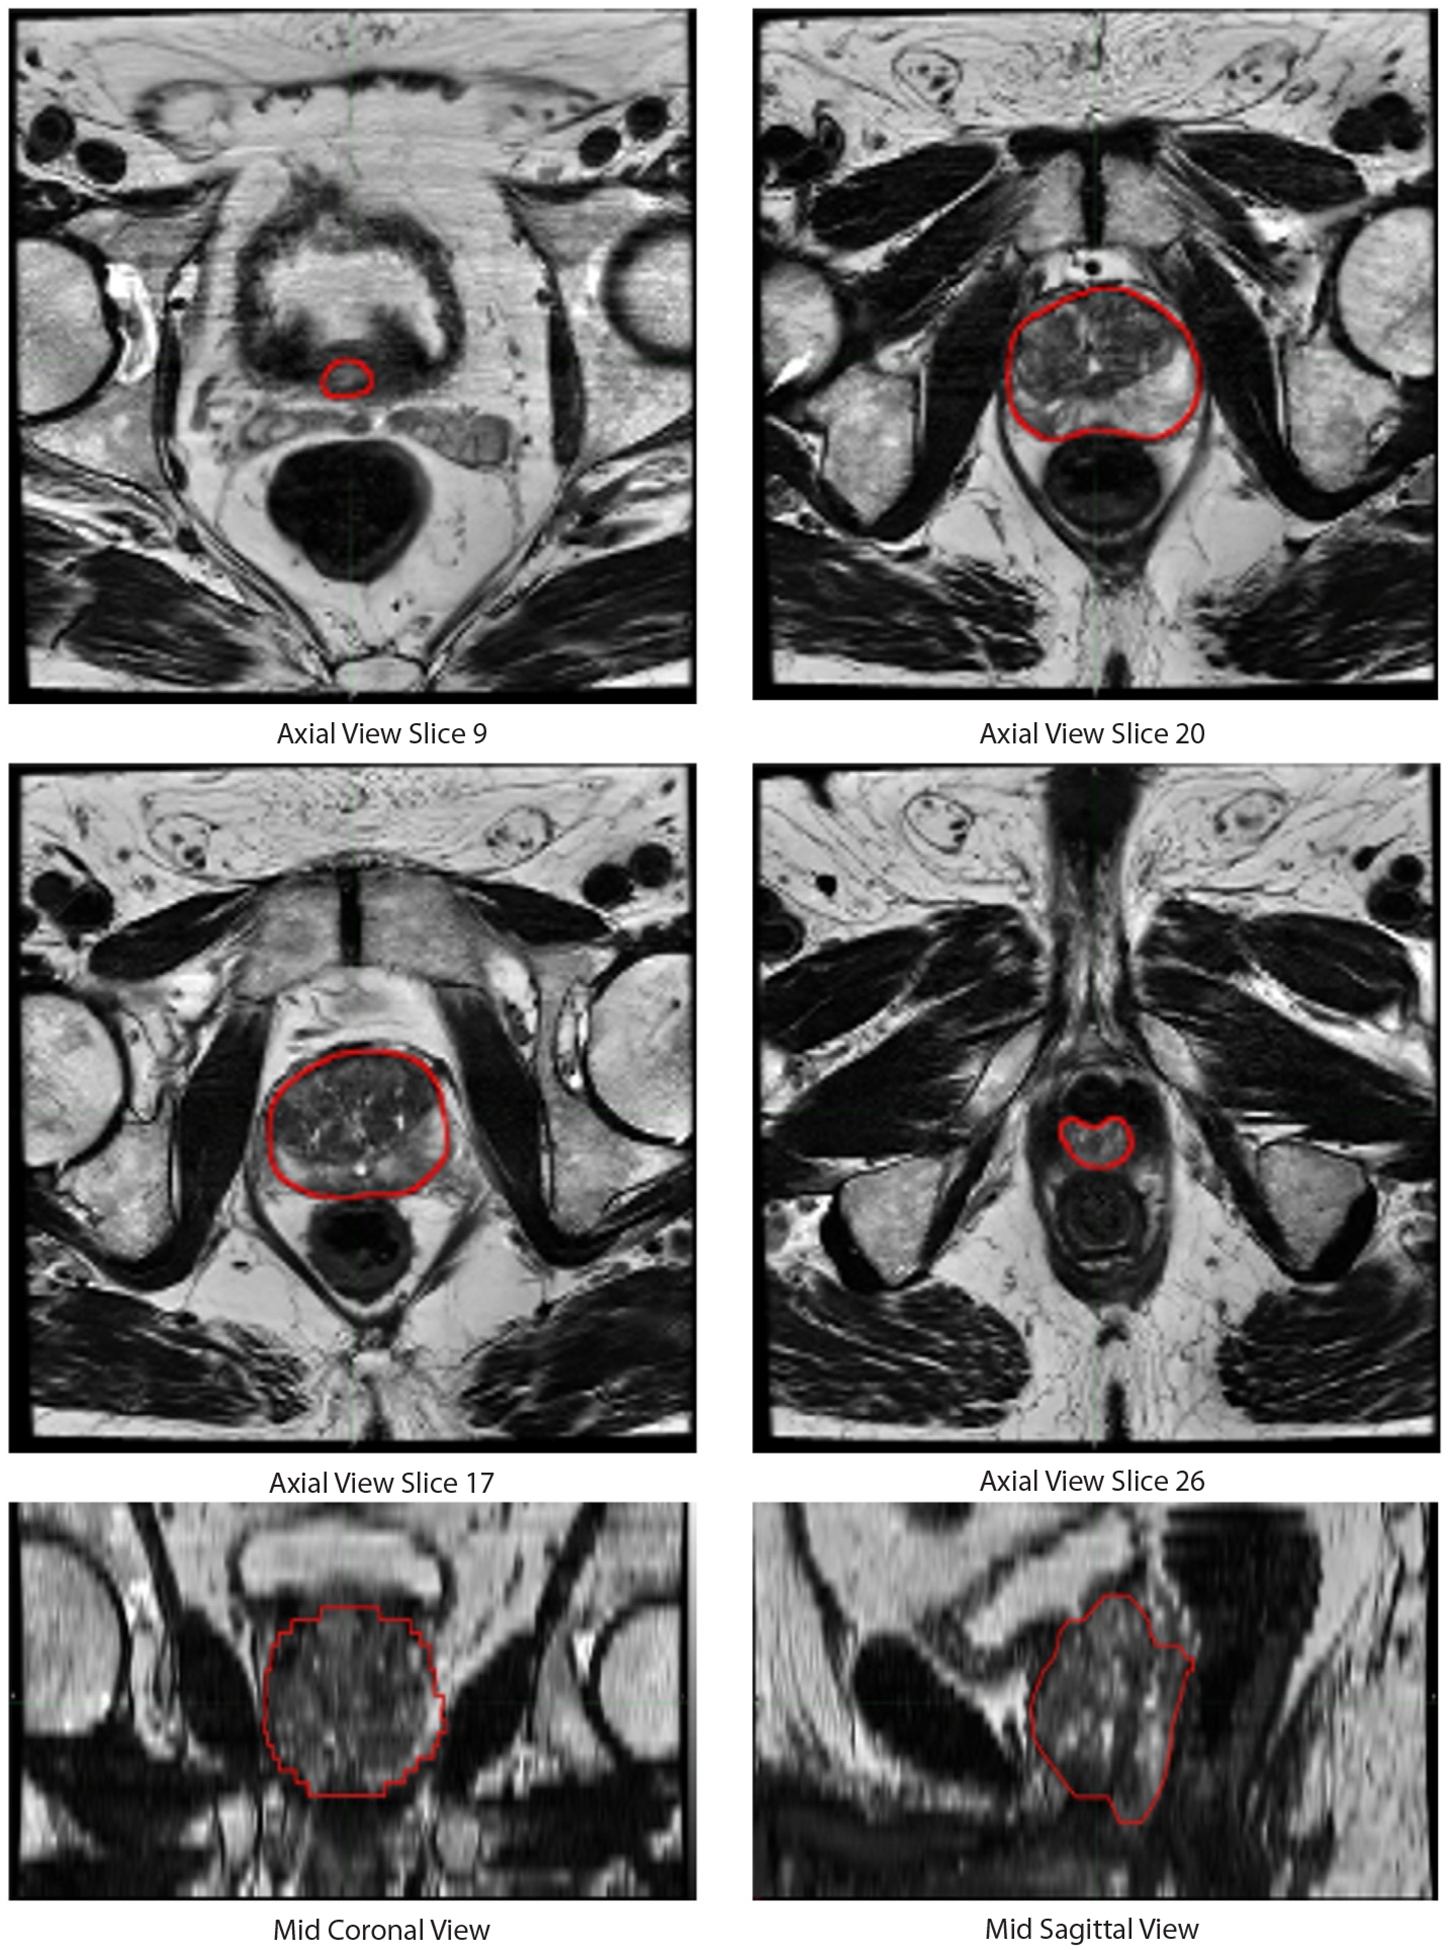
Expert-defined consensus prostate contour on magnetic resonance imaging for a representative patient case. The expert contour is shown in red contour on axial T_2_-weighted slices.
